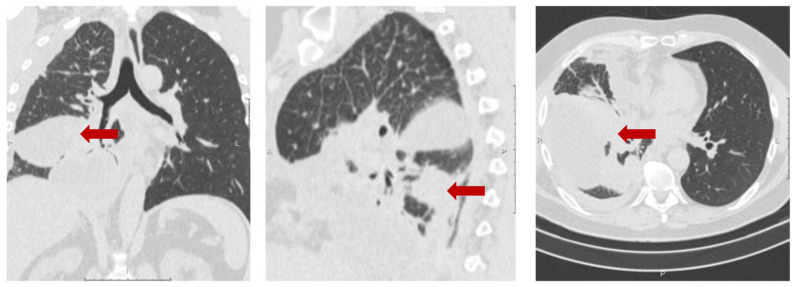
Protocol code 10: CT scan of the chest with intravenous contrast enhancement from 17 December 2020 (before the start of treatment): mass formation of the low lobe of the right lung with pre-disintegration (55 × 38 mm, along the periphery with pleural effusion 103 × 38 mm) involving the right lower lobe, intermediate bronchus and middle lobe bronchus (indicated by arrow), single small foci of the right lung up to 4 mm (mts), hydrothorax on the right with a tendency to encystment.
